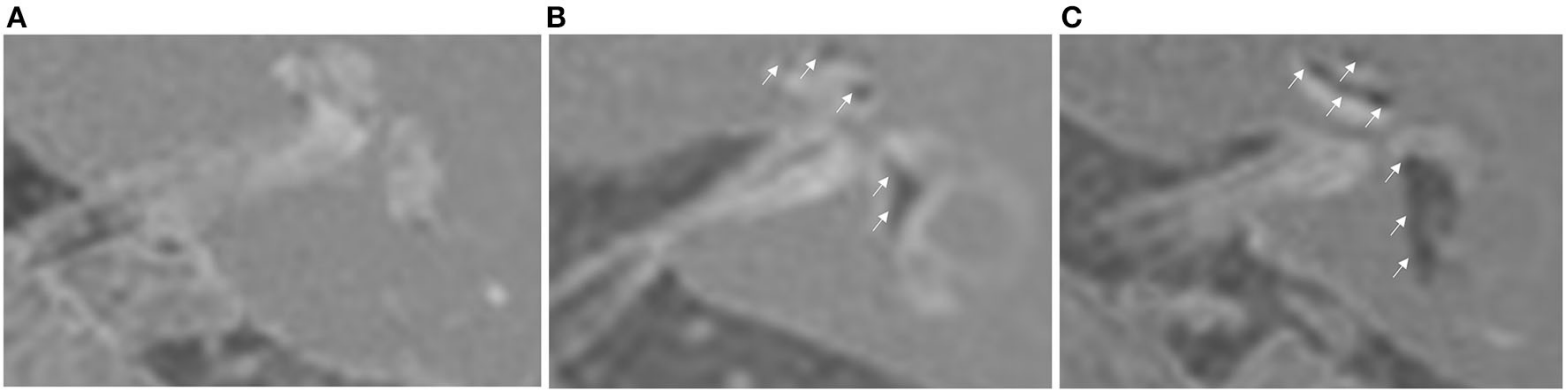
Hydrops grading based on HYDROPS MRI of the left ear. (A) Grade 0 cochlear hydrops with grade 0 vestibular hydrops. (B) Grade 1 cochlear hydrops with grade 1 vestibular hydrops. (C) Grade 2 cochlear hydrops with grade 2 vestibular hydrops.
